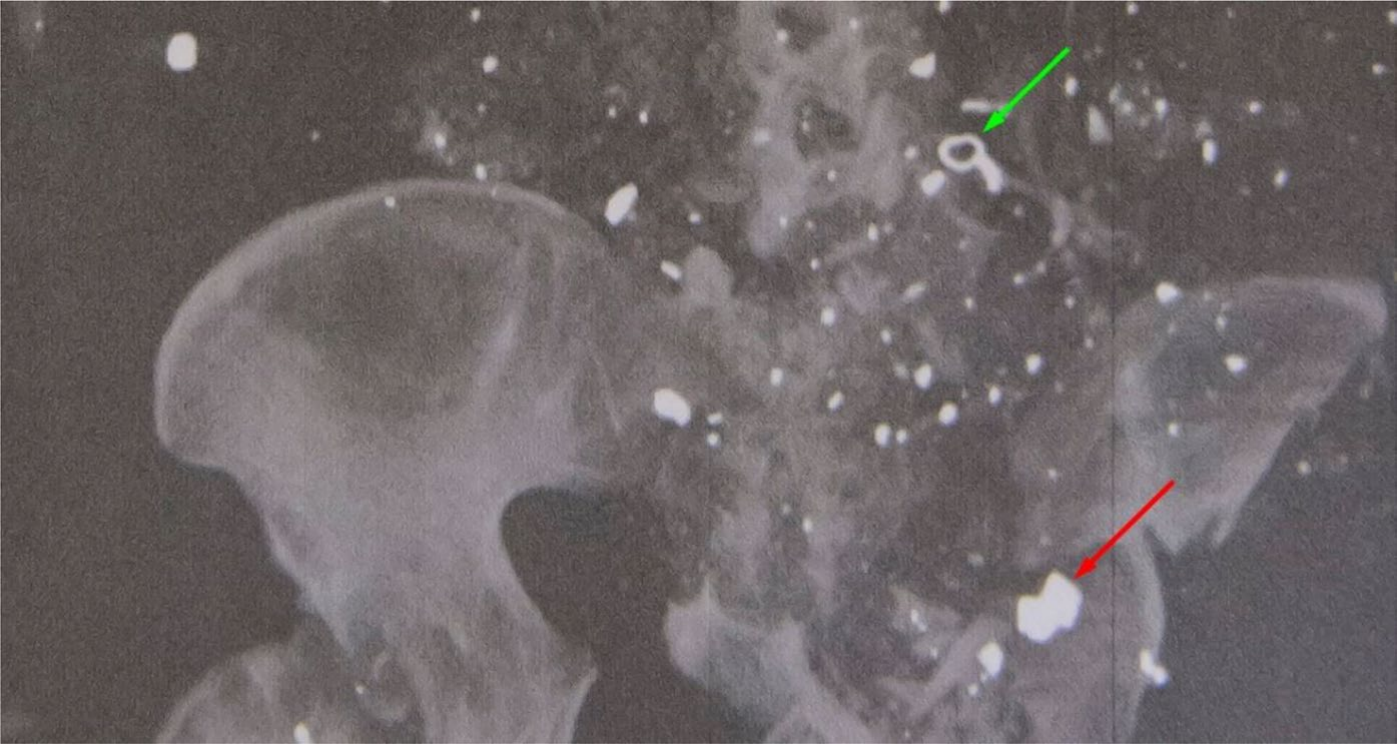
CT topogram of the remains of flight crew member #1, showing a large number of foreign objects, including a ring-shaped (green arrow) and bowtie-shaped (red arrow) fragment.
